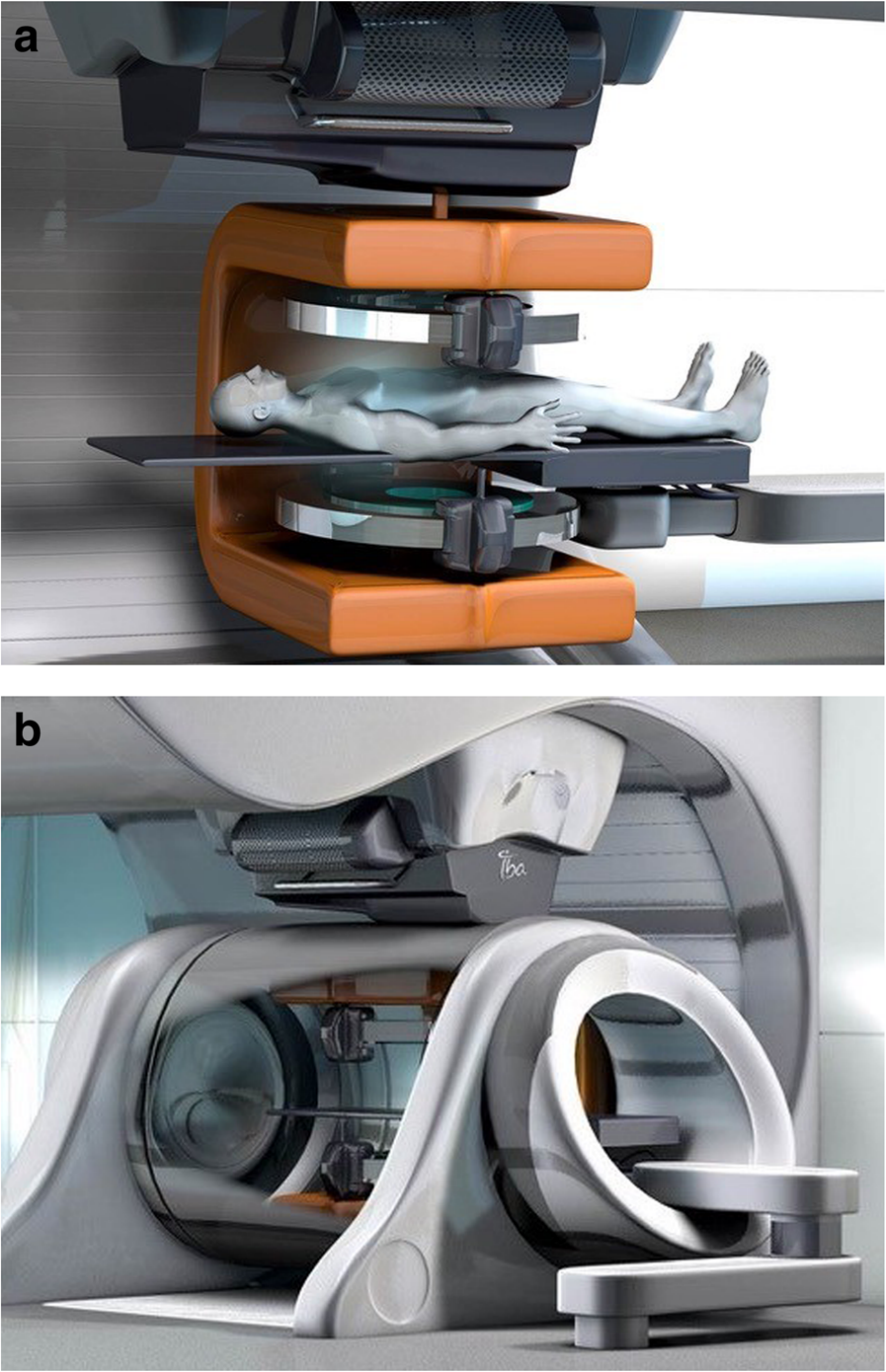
Artist impressions of (a) a rotating biplanar open in-beam MRI scanner integrated in a (b) compact proton therapy gantry treatment room (Image courtesy: Ion Beam Applications SA, Louvain-la-Neuve, Belgium)
